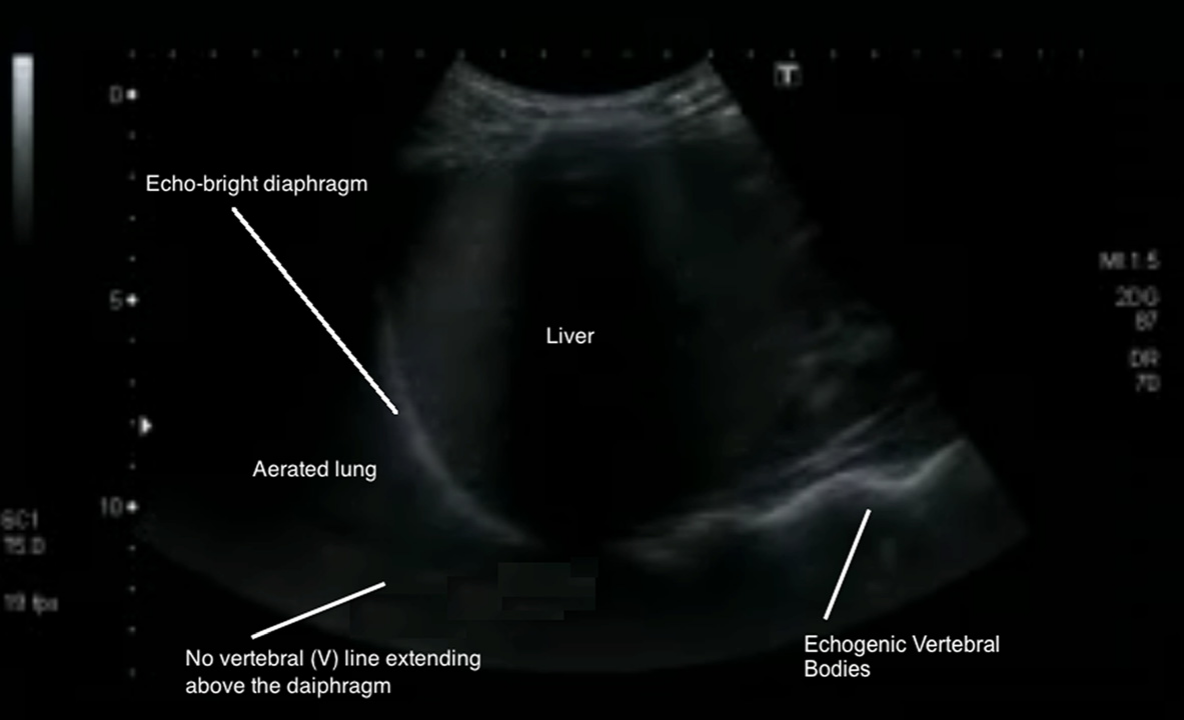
Lung ultrasound with no V line extending above the diaphragm (right lung base).
